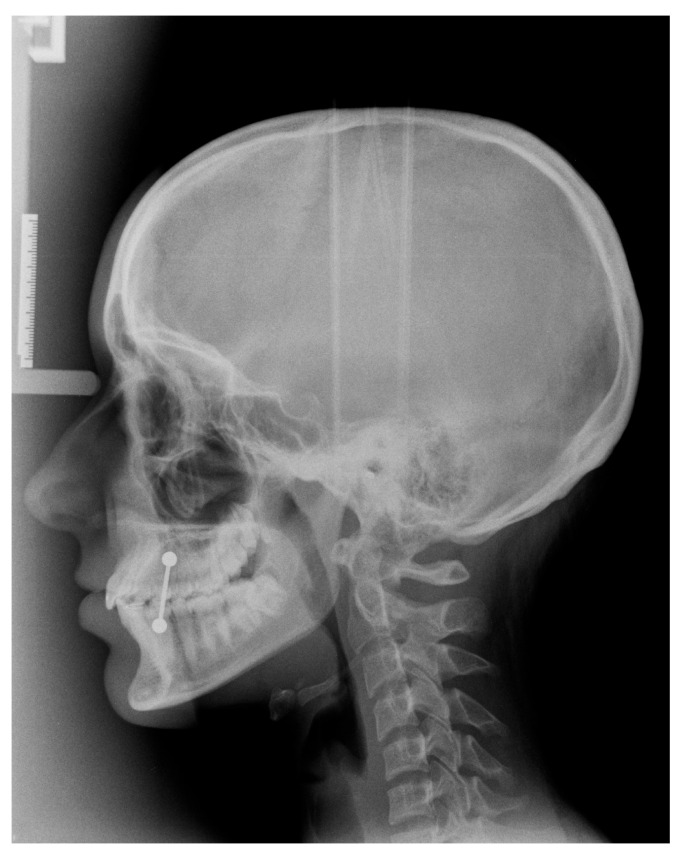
Representative lateral cephalometric radiograph acquired using an indirect digital device (photostimulable phosphor plate system), demonstrating the full craniofacial field of view, including vertex and occiput. Acquisition parameters: 90 kVp, 15 mA, 0.8 s.
